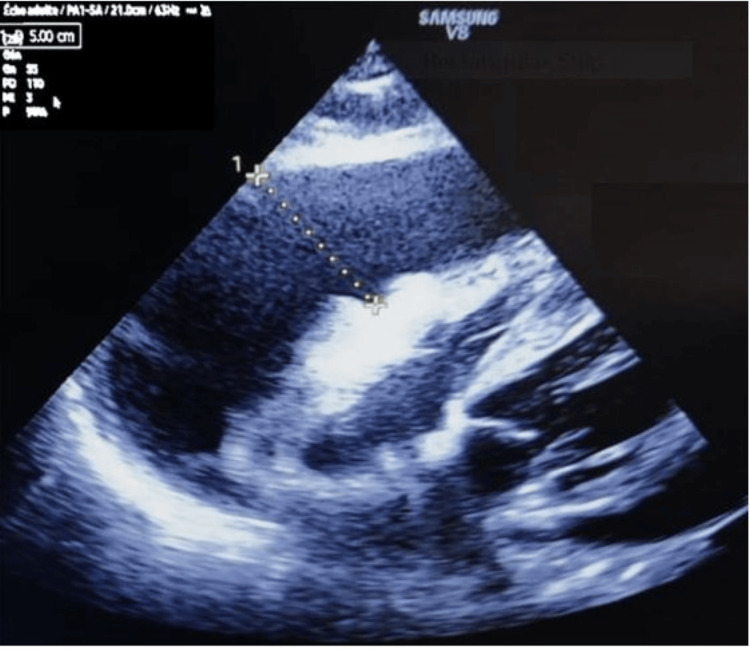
The subxiphoid (subcostal) TTE view shows a large pericardial effusion, evidenced by a significant anechoic fluid collection surrounding the heart. The measured effusion thickness is substantial, confirming severe pericardial fluid accumulation. TTE, transthoracic echocardiographic
